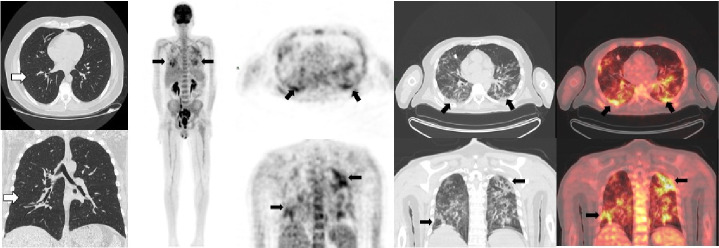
Tomografía computarizada de alta resolución de tórax sin presencia de compromiso parenquimatoso (flechas blancas). En la PET/CT F18-FDG se observó en el segmento del tórax múltiples opacidades con patrón de vidrio esmerilado, dispersas en forma de parches en ambos parénquimas pulmonares basales derechas, dominantes, con elevada actividad metabólica y SUVmáx de 7 (flechas negras).
